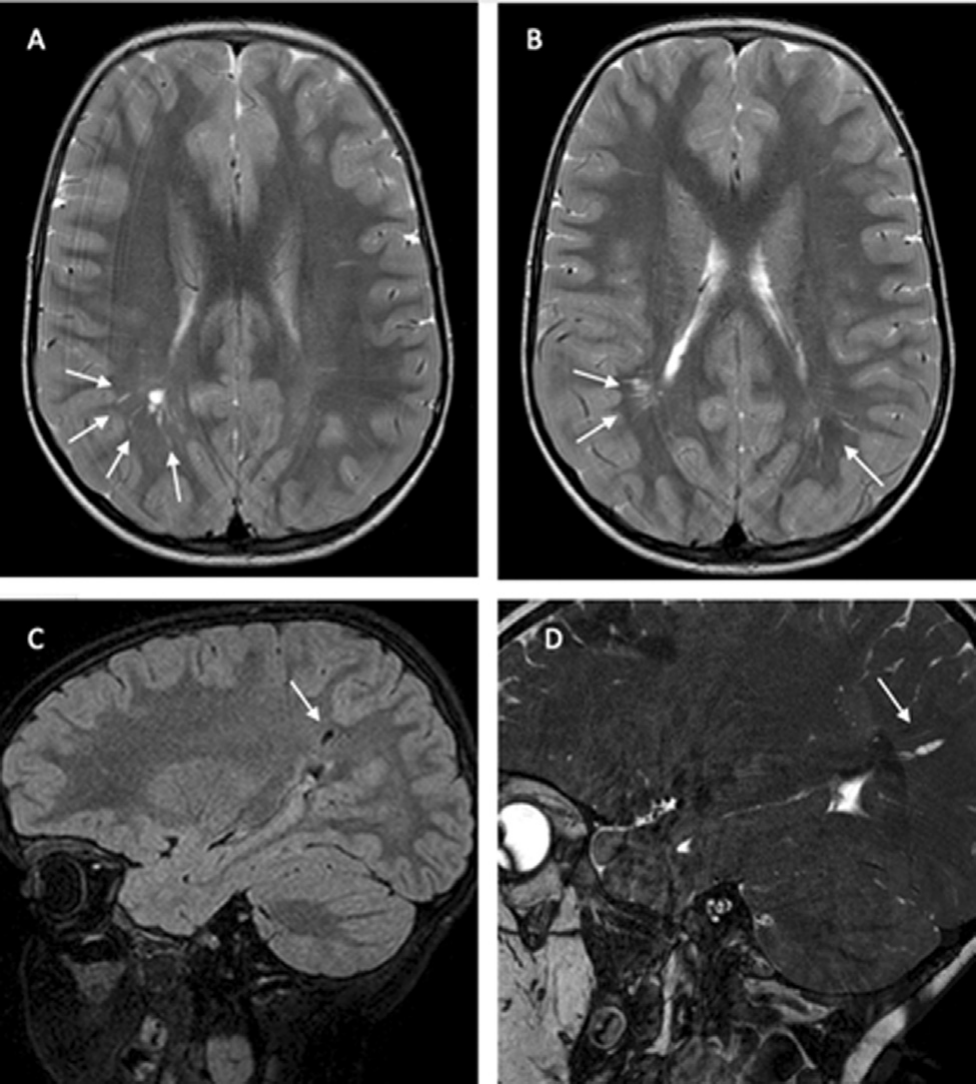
Axial T2-weighted TSE magnetic resonance imaging (MRI). (
